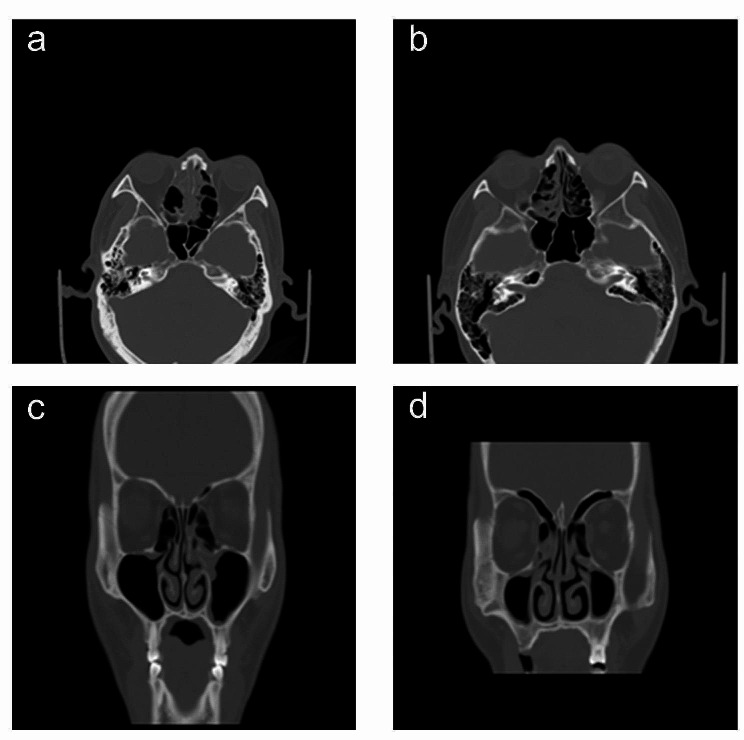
Computed tomography sections in axial (a and b) and coronal (b and d) planes of eCRS (a and c) and non-eCRS (b and d). eCRS exhibits predominant involvement in bilateral anterior and posterior ethmoid sinuses, whereas non-eCRS predominantly affects the anterior ethmoid sinuses
